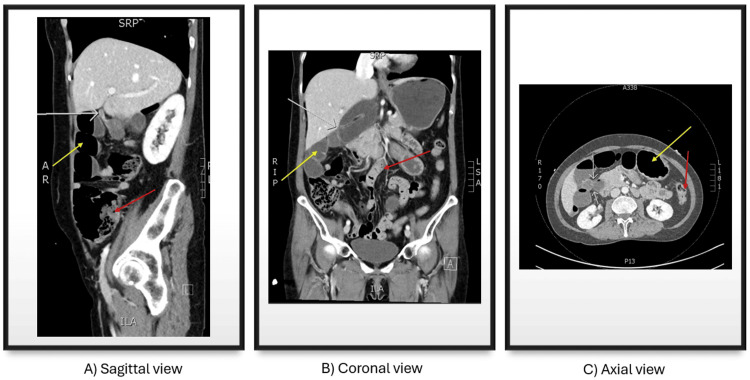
CT scan demonstrating sagittal (A), coronal (B), and axial (C) views of the herniating loop of the small bowel into the epiploic foramen (of Winslow) with abrupt transition point (marked with white arrows), proximal bowel dilatation (marked with yellow arrows), and distal collapsed loops of the bowel (marked with red arrows). CT: computed tomography
